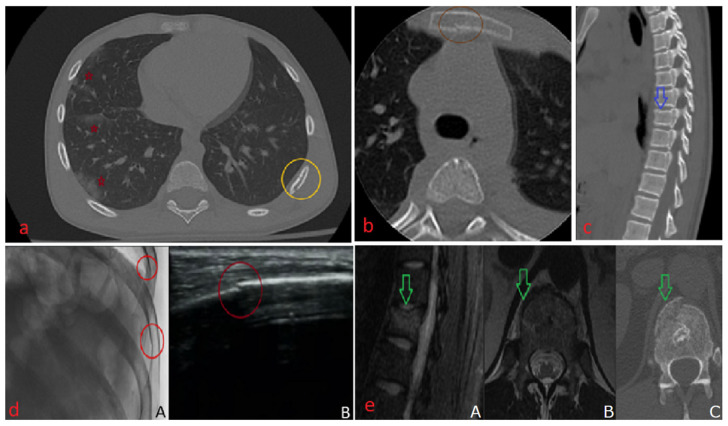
(a)—Chest CT, bone window, axial section shows two cortical disruptions of the left eighth rib arch (yellow circle), along with right pulmonary contusions—red stars; the patient had a left-sided flail chest. (b)—Spine CT, bone window, axial section reveals a unicortical disruption at the level of the sternum—orange circle. (c)—Spine CT, bone window, sagittal section reveals a compression fracture of the T8 vertebral body (blue arrow). (d)—(A). Rib series radiograph (inverted) shows cortical rib disruptions at the sites indicated by the red circles. (B). Ultrasound examination of the same patient demonstrates, within the red circle, a cortical disruption at the rib level. (e)—Green arrow shows (A). Sagittal MRI reconstruction; the STIR sequence shows a T12 compression fracture (~20% height loss), with a fracture line and STIR hyperintensity of the vertebral body consistent with bone marrow edema in an acute fracture. (B). The same fracture on axial T2-weighted MRI. (C). The fracture on CT, bone window.
